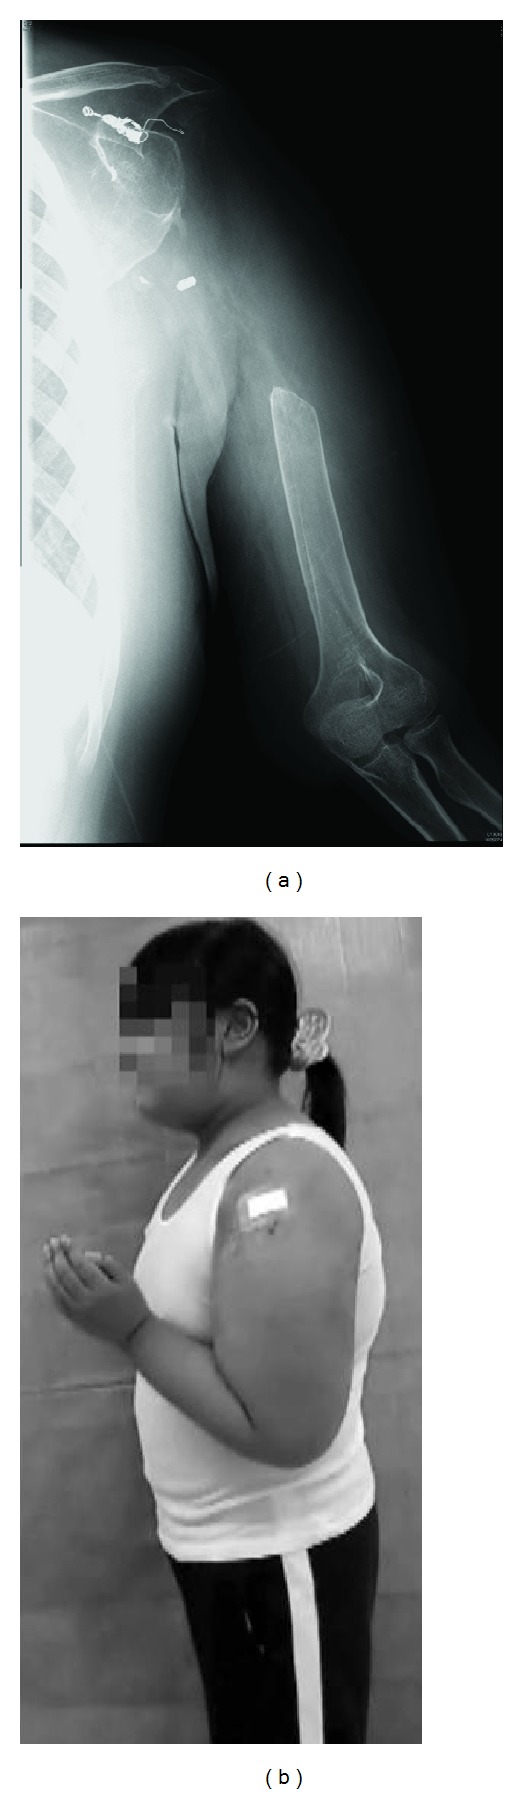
One-year postoperative radiograph showing the proximal humerus was resected and coils for preoperative embolization are left (a). Postoperative appearance of the left arm showing active elbow flexion (b).
